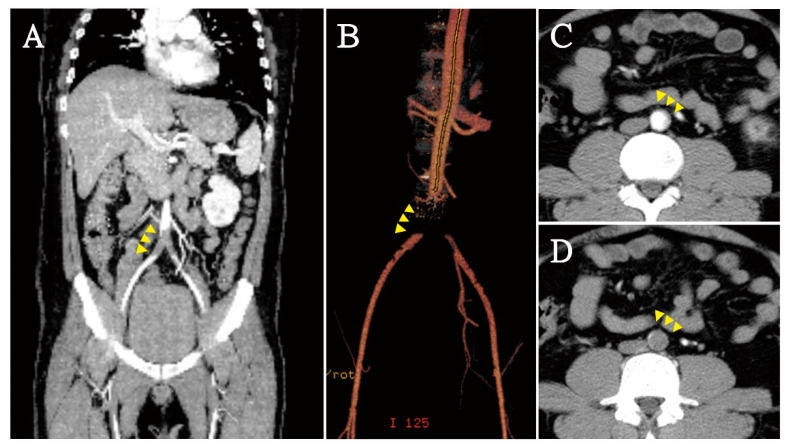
In computed tomography angiography (CTA), saddle embolism (yellow arrowhead) of distal aorta and bilateral common iliac arteries was found (A,B) in coronal plane; (C) partial occlusion at infrarenal aorta; (D) total occlusion in distal aorta.
